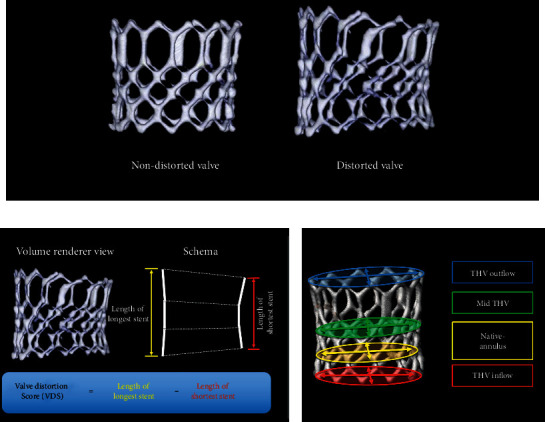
Transcatheter heart valve distortion and multislice computed tomography analysis. A normal expanded transcatheter heart valve (THV) and distorted THV in a multislice computed tomography (MSCT) volume-rendered view (a). Using the postprocedural MSCT, a 3D-volume-rendered view of THV was constituted. The length of the stent frame on the center of each cusp was measured. Valve distortion score (VDS) was defined as the difference between the longest and shortest stent frame lengths (b). The THV stent frame was geometrically evaluated for maximal and minimal orthogonal diameters at the four levels of THV: THV inflow, native annulus, mid-THV, and THV outflow. The cross-sectional eccentricity index was defined as follows: (1 − short diameter/long diameter) × 100 (c).
